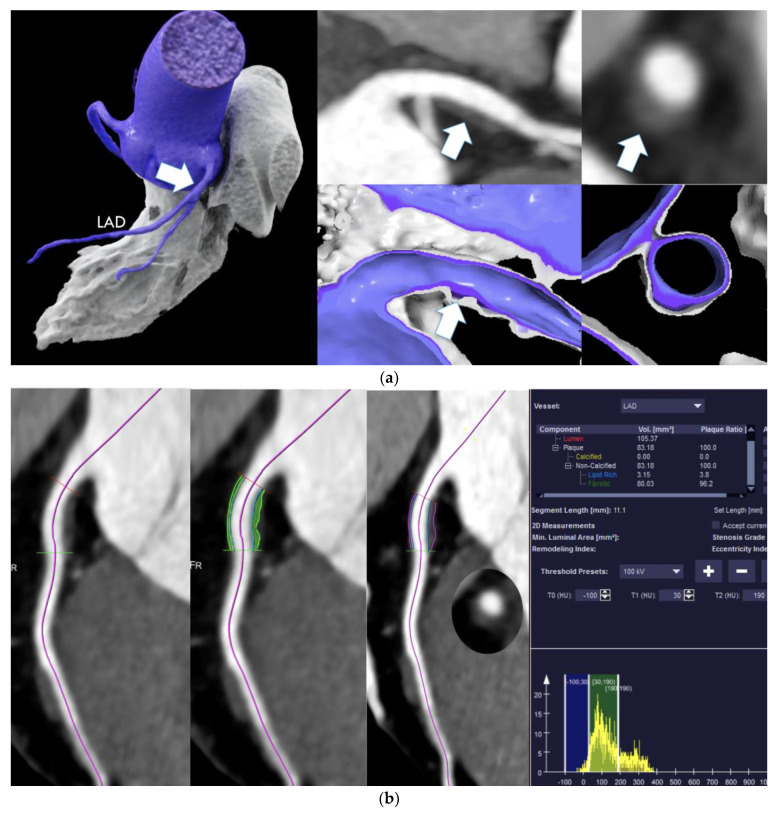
(a) A 30 year-old-male with diabetes since 14 years, presenting with ketoacidosis to our ED. Hs-Troponin (49 ng/dL) and HBA1c (11.2%) were elevated. CTA (A) showed a non-calcified lesion (white arrows) in the proximal LAD with positive remodeling (“high-risk- plaque”—HRP) with quantitative 3.15 mm^3^ lipid-rich necrotic core plaque volume (LAP < 30 HU) (b) based on CTA. CACS was 0. There was no calcific plaque components by CTA (a). Manual contour editing (b) was performed if automated tracing of lesion borders was inaccurate (mid cMPR and right cMPR) (SyngoVIA^TM^ Frontier Research, Siemens Healthineers): VRT (left) and MPR (mid and right). (b) cMPR and quantitative plaque analysis.
